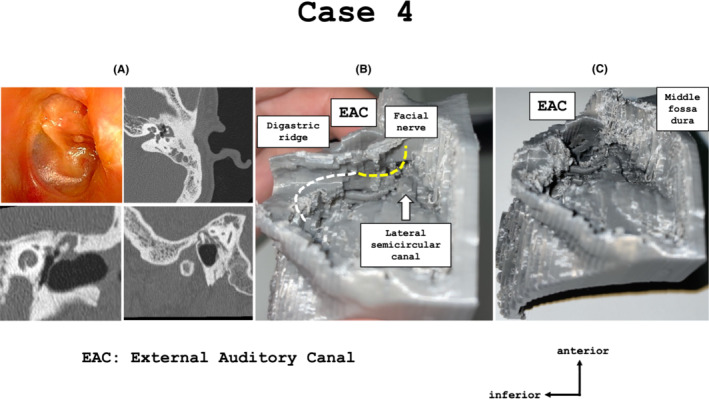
(A) In case 4, tympanoplasty type III and canal wall down mastoidectomy were performed for left flaccid‐type cholesteatoma. The computed tomography sagittal view showed that the middle fossa plate was lower towards the anterior, and the cholesteatoma extended to the mastoid and supratubal recess from the attic. (B) A full‐scale model that was performed the canal wall up mastoidectomy was created. We confirmed the line of the sigmoid sinus, posterior wall of the external acoustic canal, digastric ridge, facial nerve, and lateral semicircular canal. (C) We created the model that was used to performed the canal wall down mastoidectomy. We confirmed that the anterior of the middle fossa plate was lower.
